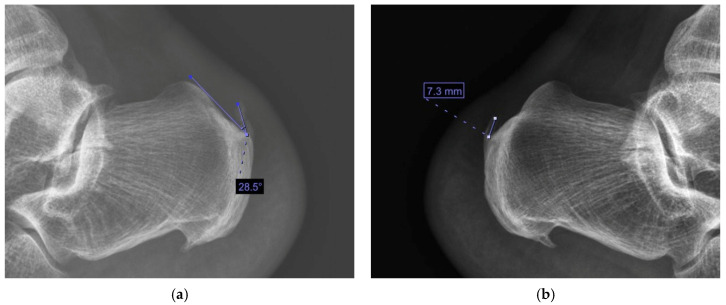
Radiographic measurement of posterior calcaneal spur morphology. (a) Spur length was defined as the maximal linear distance (in millimeters) measured from the posterior calcaneal cortex to the distal tip of the enthesophyte along the longitudinal axis of the spur. (b) Spur angle was defined as the angle formed between (i) the longitudinal axis of the enthesophyte and (ii) a reference line drawn tangential to the posterior calcaneal cortex at the base of the spur.
